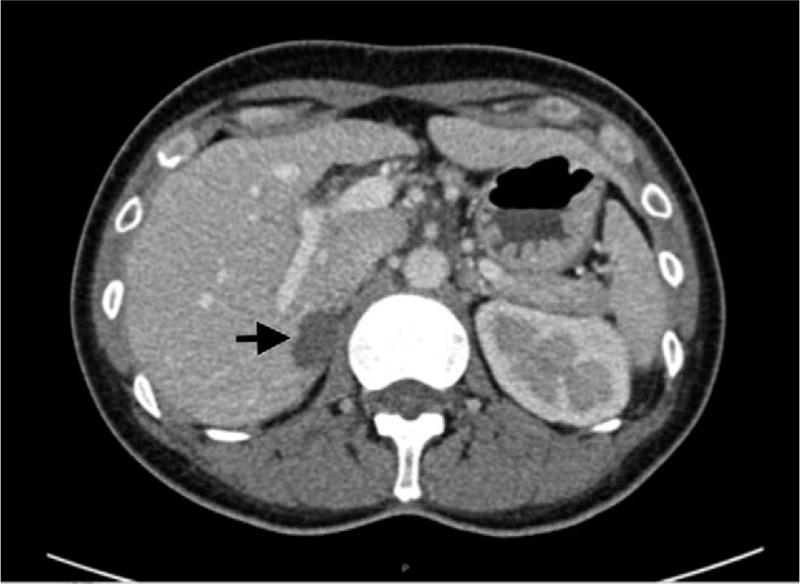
Contrast abdominal CT showing a mass lesion (22 mm) of right adrenal gland (arrow). CT = computed tomography.
